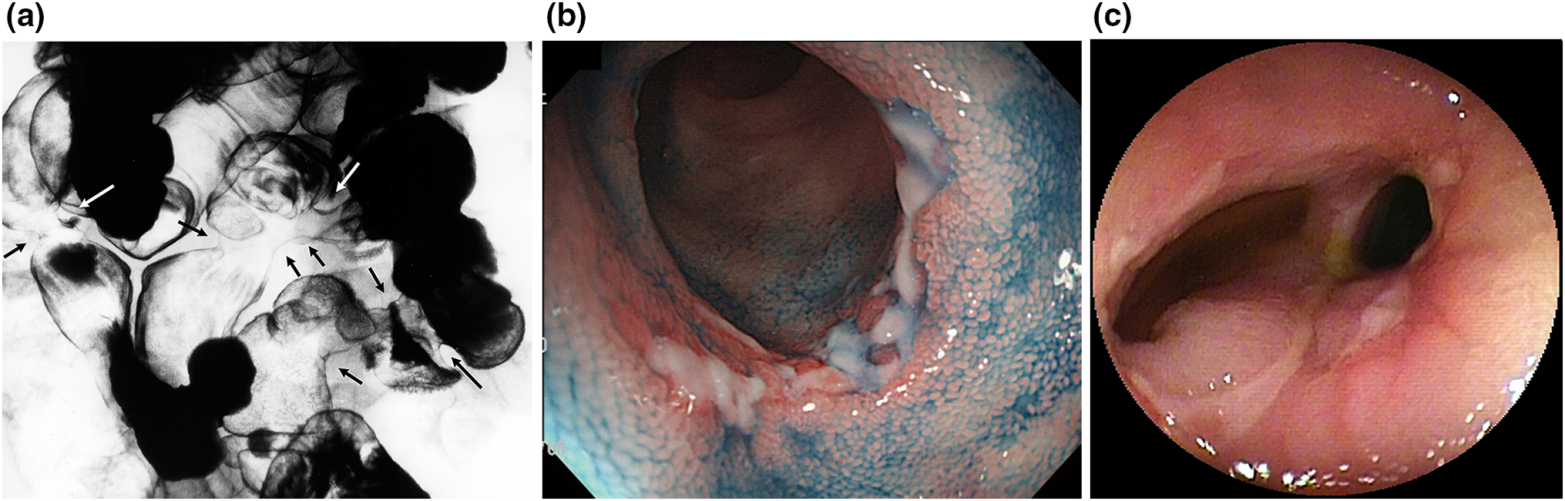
Radiographic and endoscopic findings of small intestinal lesions. a Double-contrast radiography depicts multiple deformities and strictures at the distal jejunum and ileum (*arrows*). b, c Endoscopic findings. Shallow circular ulcers (b) or circular and oblique ulcers with symmetrical deformity and pseudodiverticulum formation (c) in patients with CEAS. Figures are reprinted with permission from Refs. [10]
